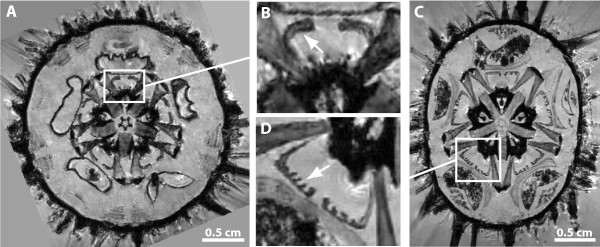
Virtual horizontal sections of 2D MRI scans of whole sea urchins (A, C) reveal distinct shapes of protractor muscles in *Paracentrotus lividus *(B) and *Echinometra mathaei *(D) (arrows). Magnetic resonance imaging was carried out in Berlin, Germany using a high-field MRI scanner with a 7 T super-conducting electromagnet (Bruker Biospin GmbH, Ettlingen, Germany). Image processing of the ~10 MB large raw image datasets was carried out using ImageJ 1.42q (NIH, Bethesda, USA) and its Volume Viewer plug-in on a standard office PC. The sea urchin (Echinodermata: Echinoidea) species shown here were collected in the wild (*Paracentrotus lividus*) or taken from a museum collection (*Echinometra mathaei*, NHM 1969.5.1.61-75).
